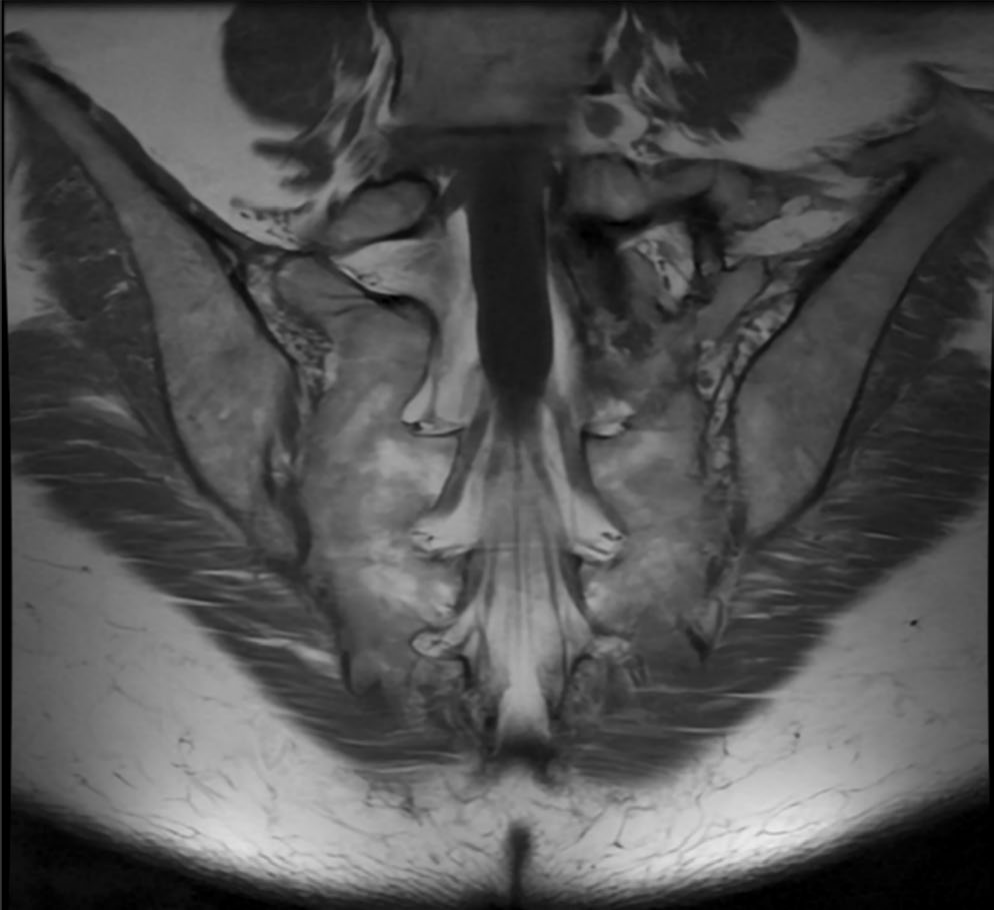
MRI of bilateral sacroiliac joints. Demonstrating partial sacralization of the left L5 vertebral body with hypertrophic pseudoarticulation with the left sacral ala. Degenerative changes of the right sacroiliac joint noted.
